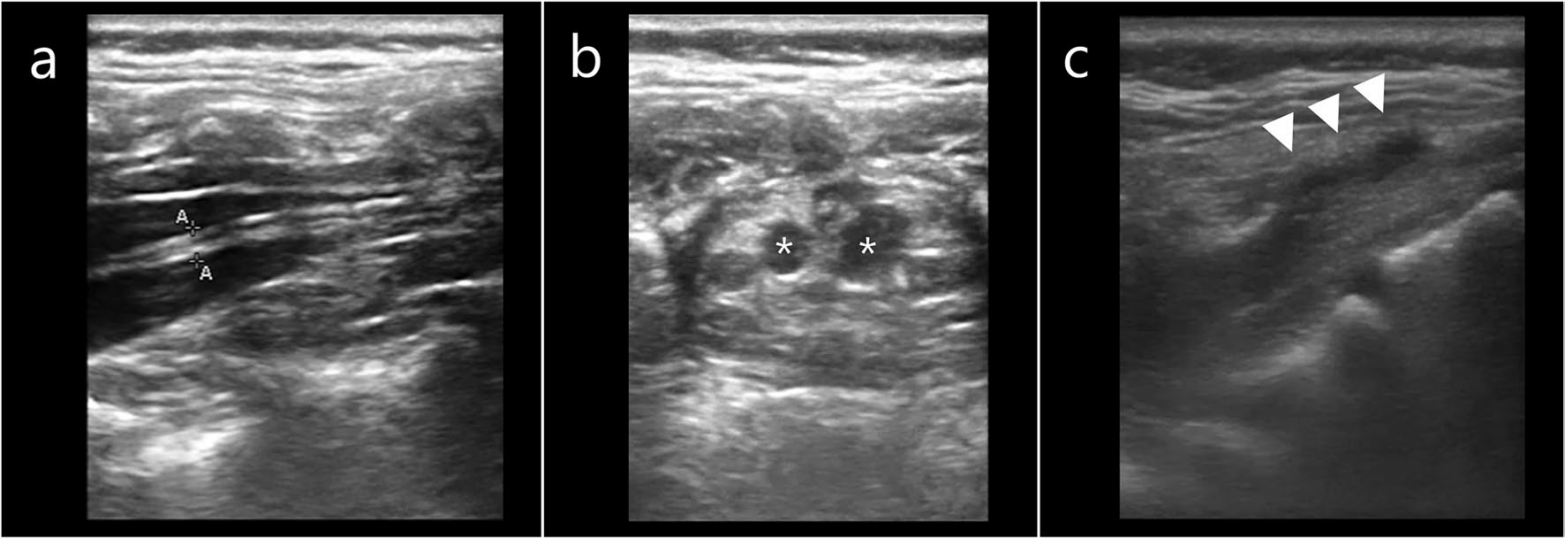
Spinal abnormalities identified on spinal ultrasound. a Spinal ultrasound of a 22-month-old child with filum terminale lipoma. The filum terminale is 2.0 mm thick and surrounded by hyperechoic fatty tissue. b Spinal ultrasound of a 12-month-old child showing Tarlov cysts (*). c Spinal ultrasound showing a low-lying dural sac at the S4 level, continuous with a dermal sinus (arrows)
